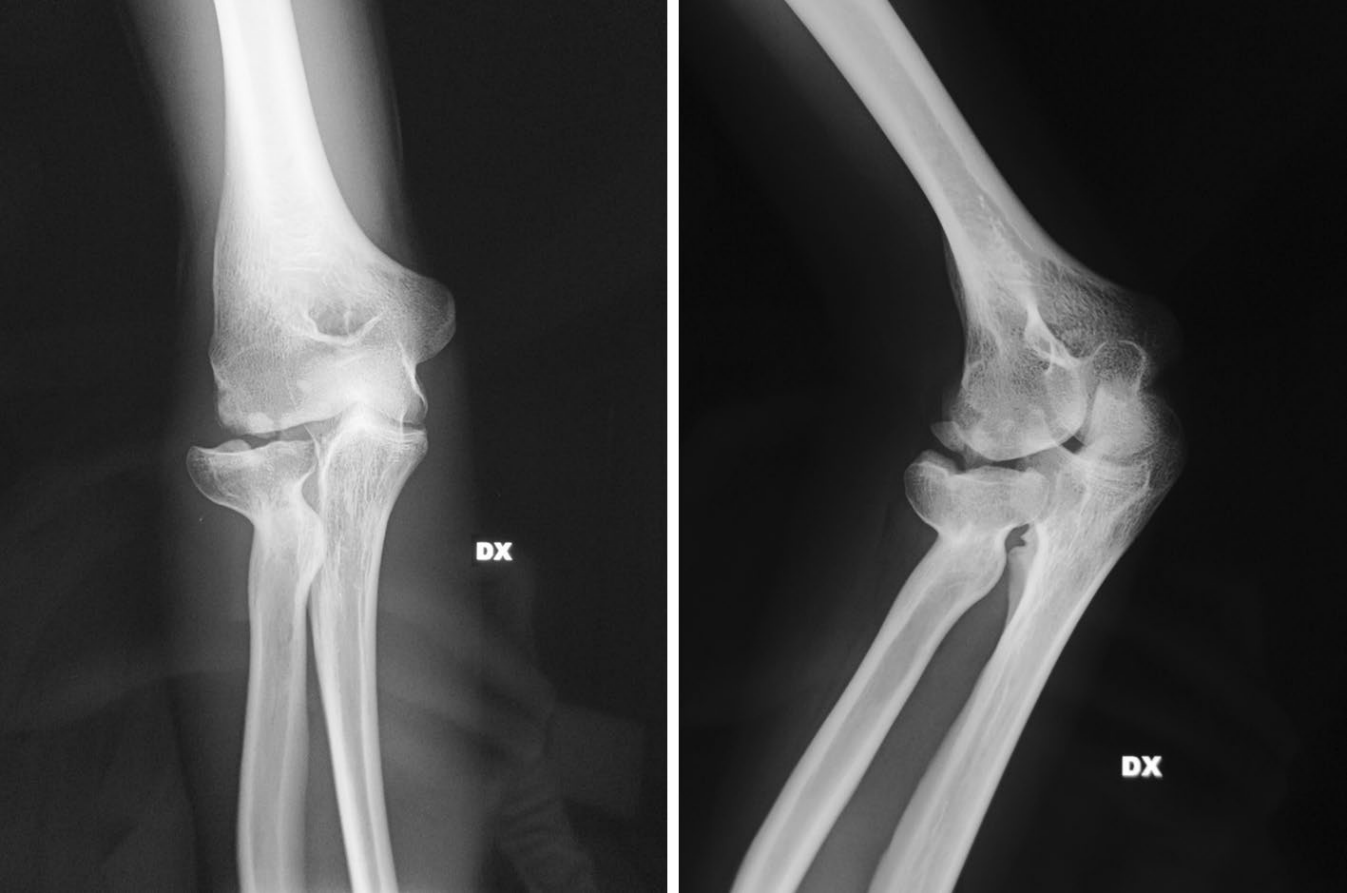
​Pre-op X-rays of 18-year-old boy with radial head malunion, calcifications and secondary humeroradial arthritis
